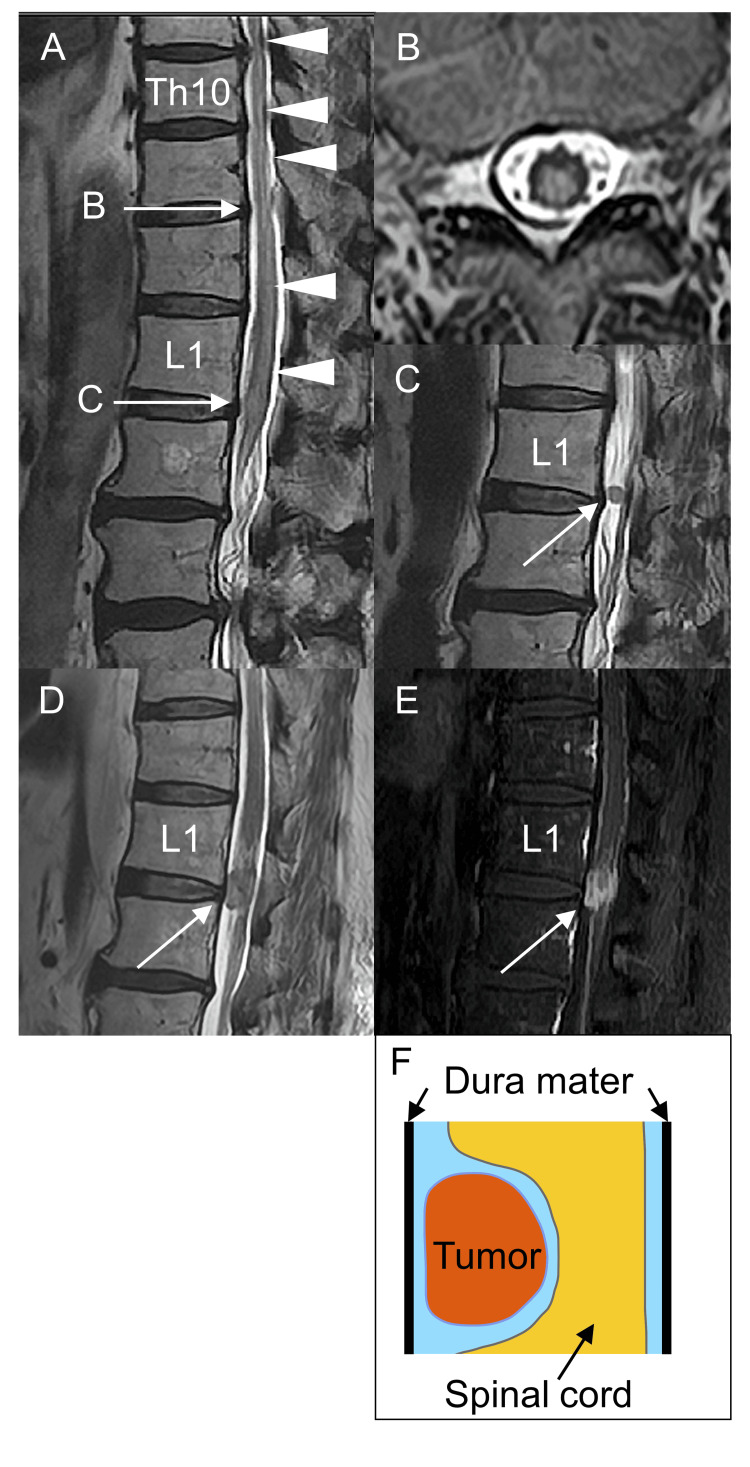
Intradural extramedullary spinal metastases with myelitis. A: sagittal T2-weighted magnetic resonance image (MRI) shows an intramedullary long spinal cord lesion from Th10 to L2 spine (arrowheads); B: axial T2-weighted image at the Th11 level shows widespread high-intensity signals in the spinal cord; C: sagittal T2-weighted image at the L1 level shows an intradural extramedullary lesion slightly to the right of center (arrow); D: despite treatment with alectinib, the intradural extramedullary metastasis lesion at the L1 level became enlarged (arrow); E: gadolinium-enhanced T1-weighted MRI shows a growing intradural extramedullary nodule (arrow); F: representation of intradural extramedullary spinal metastases.
